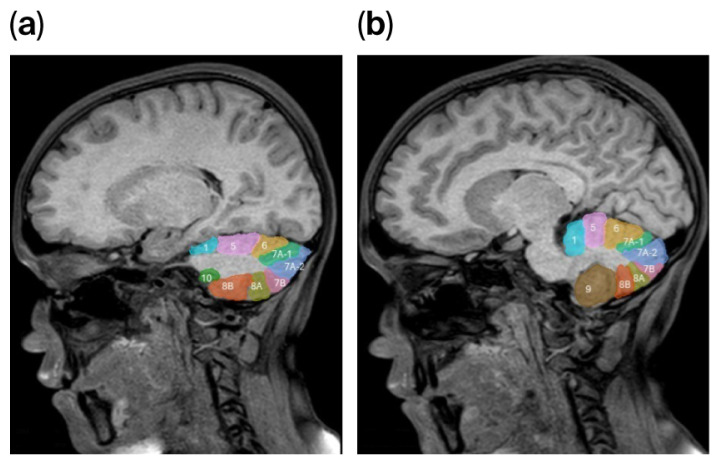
(a,b) Sagittal T1-weighted MR images showing the semi-automated segmentation of lobular anatomy in the right cerebellar hemisphere performed using 3D Slicer (version 5.6.1). Regions delineated with different color tones correspond to distinct lobular subregions defined according to Schmahmann’s cerebellar atlas. Numerical labels indicate individual cerebellar lobules, where lobule 7A-1 represents Crus I and lobule 7A-2 represents Crus II.
